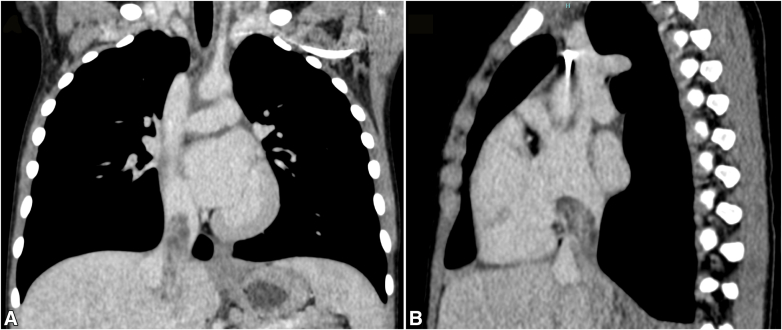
Preoperative contrast-enhanced computed tomography of patient 2. Coronal (A) and sagittal (B) images demonstrating intravascular tumor thrombus extending into the inferior vena cava and right atrium.
